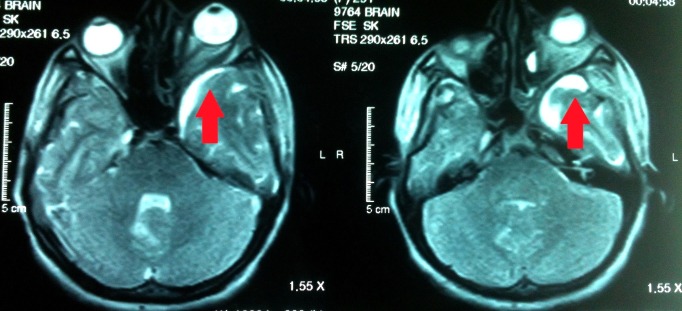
MRI T2WI showing deformity of left cerebral hemisphere causing extrinsic pressure towards left orbit leading to proptosis. MRI: Magnetic resonance imaging; T2WI: T2 weighted image.
